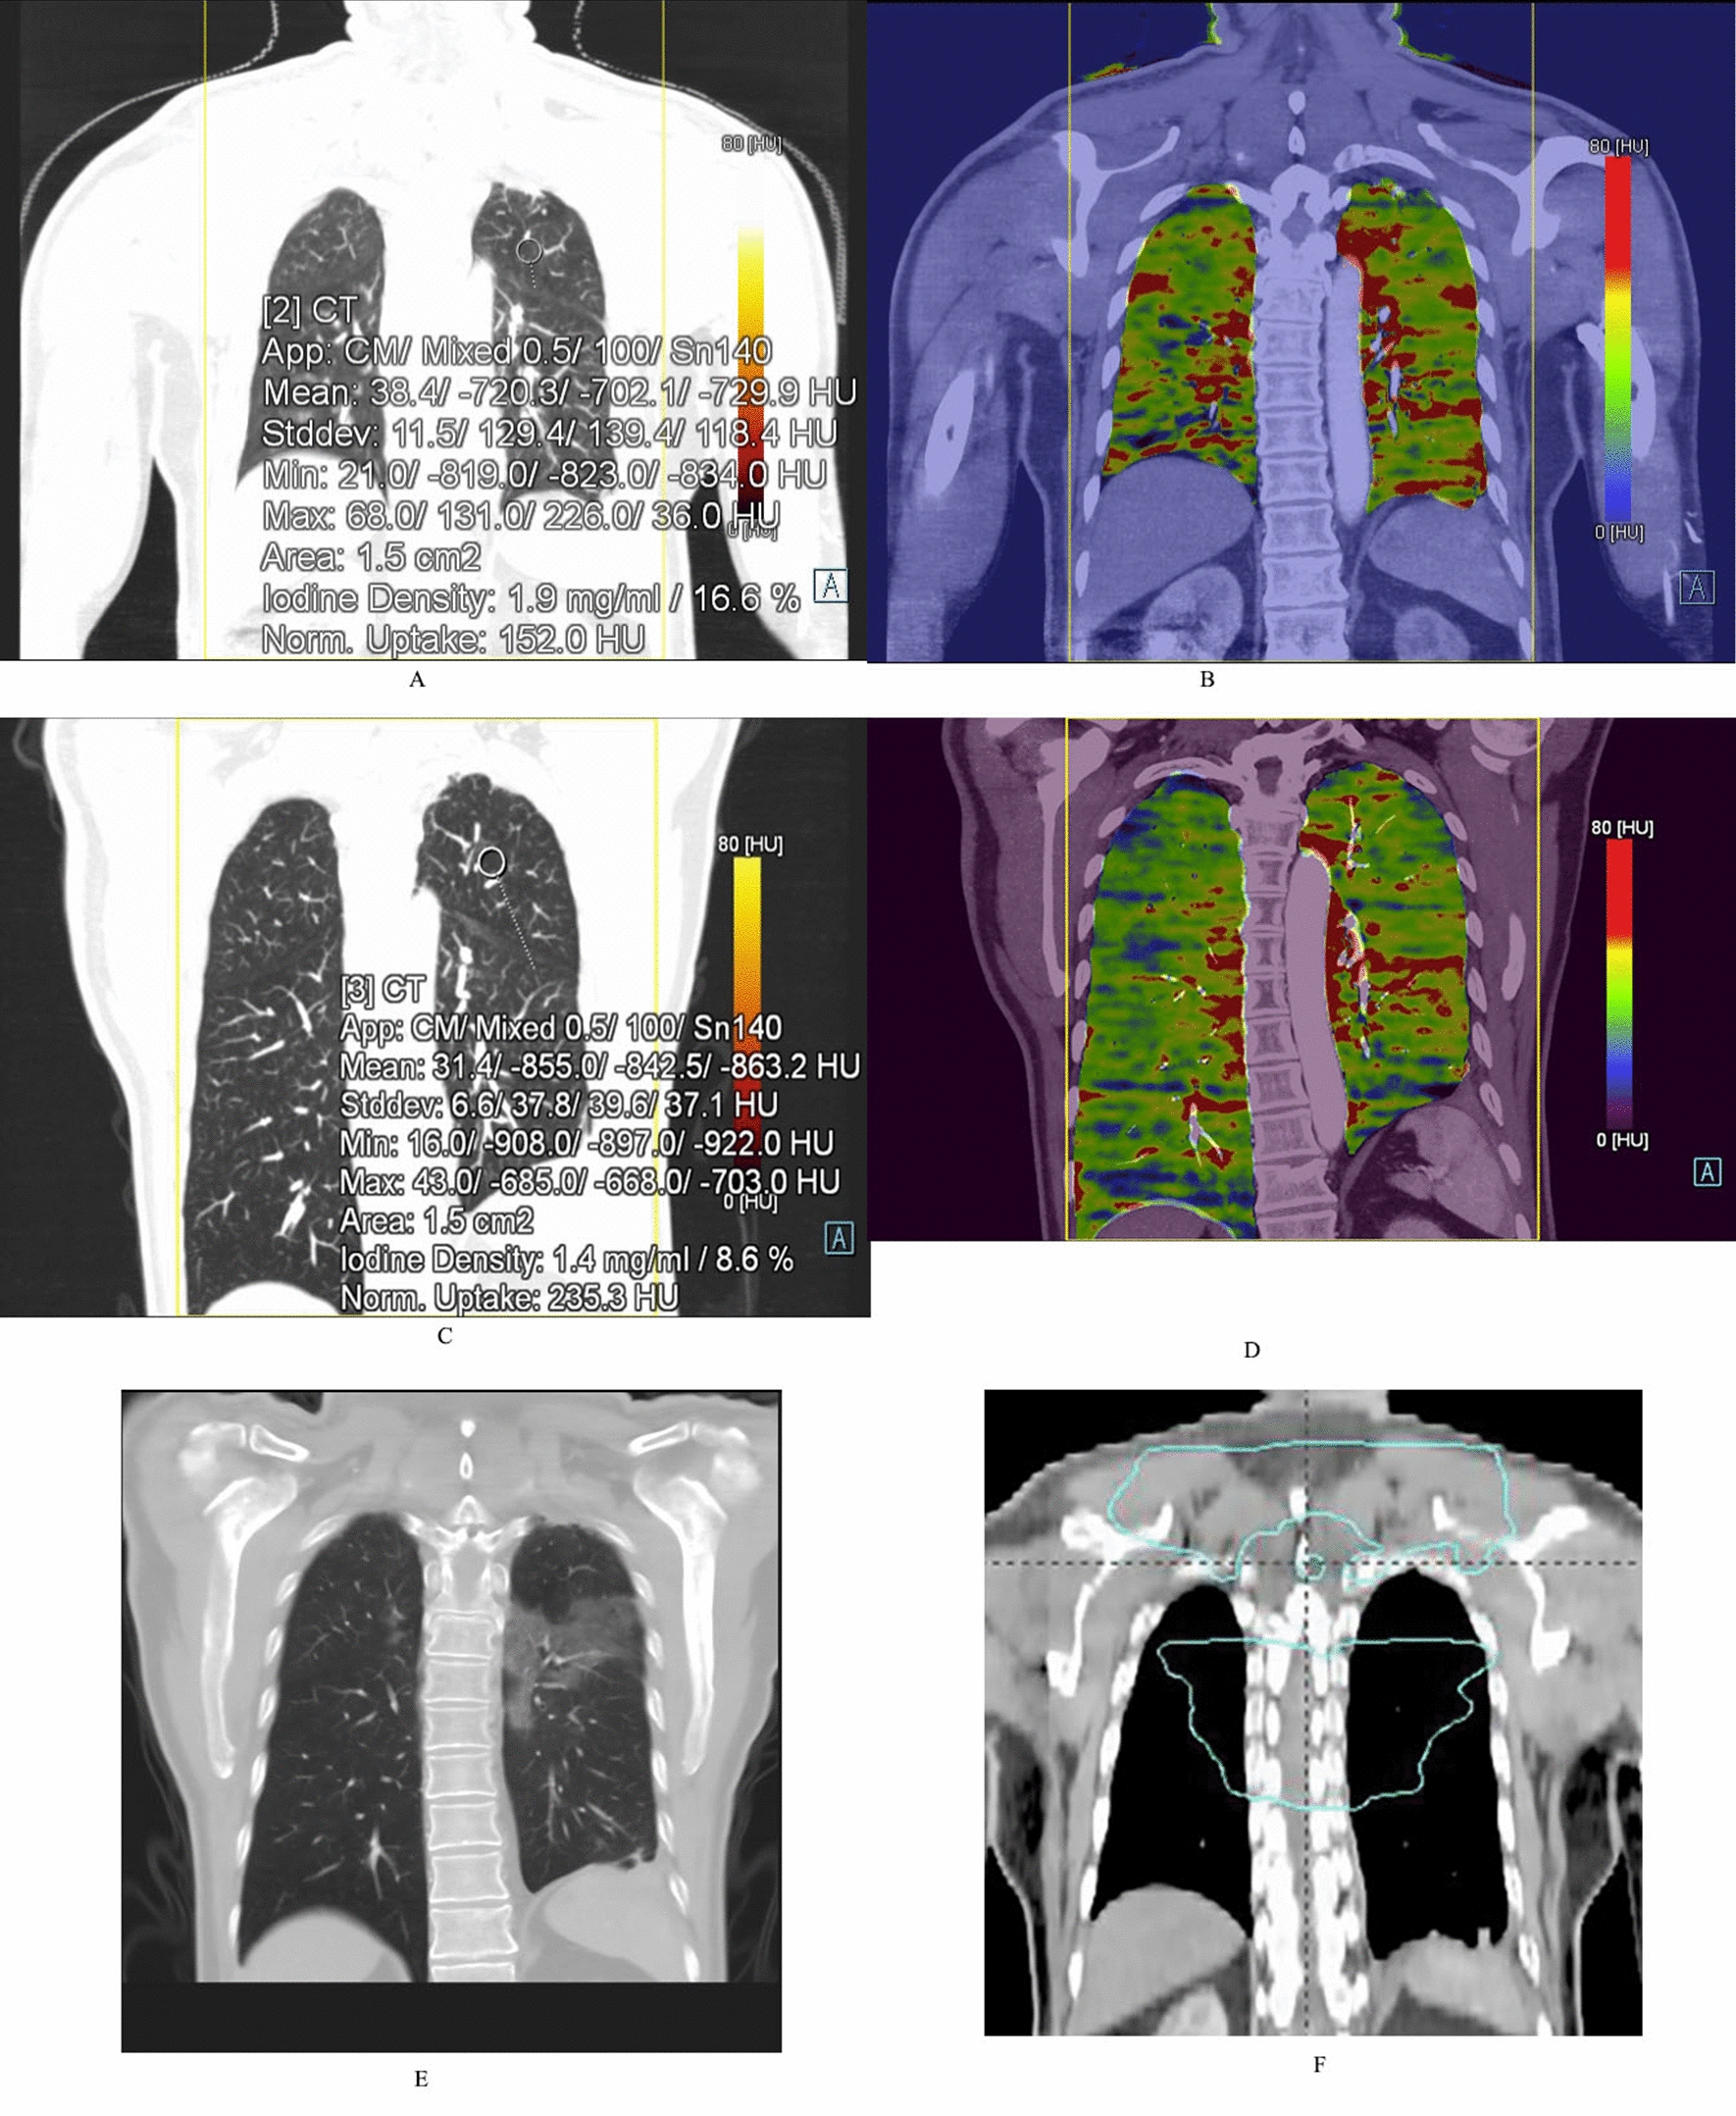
Female/63 years old, upper segment esophageal cancer; a Dual-energy lung window image before radiotherapy, the NIC value of the upper left lung is 0.166; b Lung PBV color image before radiotherapy, blood flow (red part) is more abundant in bilateral irradiated fields; c Dual-energy lung window image after radiotherapy, the NIC value of the upper left lung is 0.086; d Lung PBV color image after radiotherapy, blood flow (red part) is less than before. e CT image during follow-up period, ARP is seen in both upper lungs; f TPS coronal localization image, the region of V_>20 Gy_ irradiation is within the green line, and ARP is seen in the region
